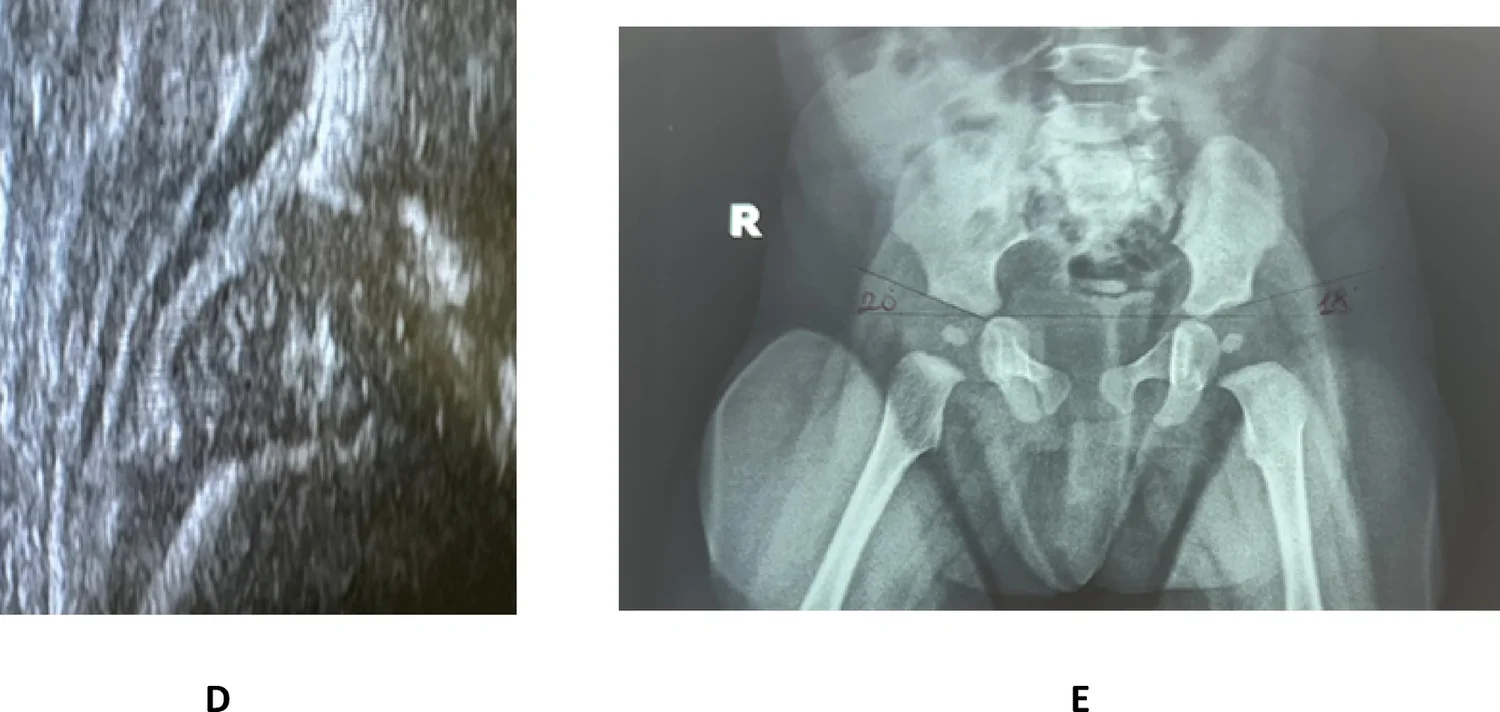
Representative clinical case showing progressive ultrasonographic and radiographic normalization during Coxaflex treatment. A: Female, 18 days of age. Born at term, from a spontaneous delivery and with no family history of DDH. Positive Ortolani test on the left side.She therefore underwent hip ultrasonography according to the Graf method, which showed a type 2 C hip on the left with an α angle of 49° and a β angle of 60° (T0). Patient was therefore immediately treated with a Coxaflex brace full-time, with careful parental education regarding its use (worn 23 h/day). B 50 days Follow-up (T1): Improvement in the Graf angles, with an α angle of 60° and a β angle of 55°. The ossification nucleus of the femoral head was also visible. C Follow-up performed at 90 days (T2) αangle = 67°, while the β angle = 55°. D 120 days follow-up (T3) US stabilization of the Graf angle values within the normal range ( α angle = 69° and β = 55°)( Fig. 2D). Radiographic follow-up performed at 120 days (Fig. 2E). The radiograph showed, on the affected left side, an acetabular index (AI) of 18°, within the normal percentile range for age and sex (< 25°) and comparable to the contralateral side (AI 20°). After normalization of ultrasound and radiographic parameters, the patient discontinued the Coxaflex brace.
